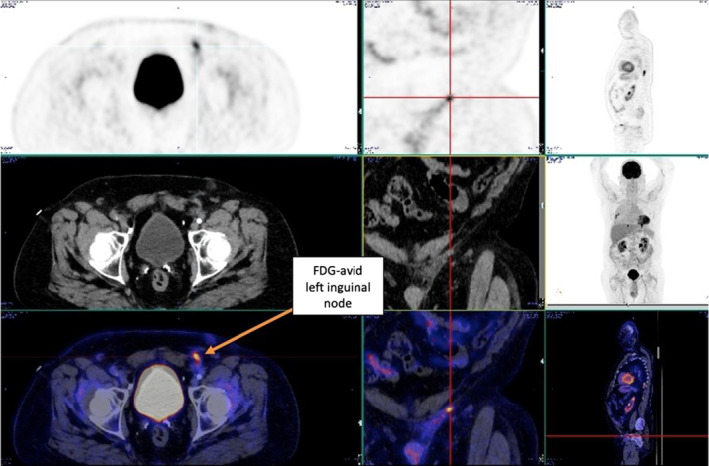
FDG‐avid residual left inguinal node post orchiectomy, suggestive of metastatic disease.
